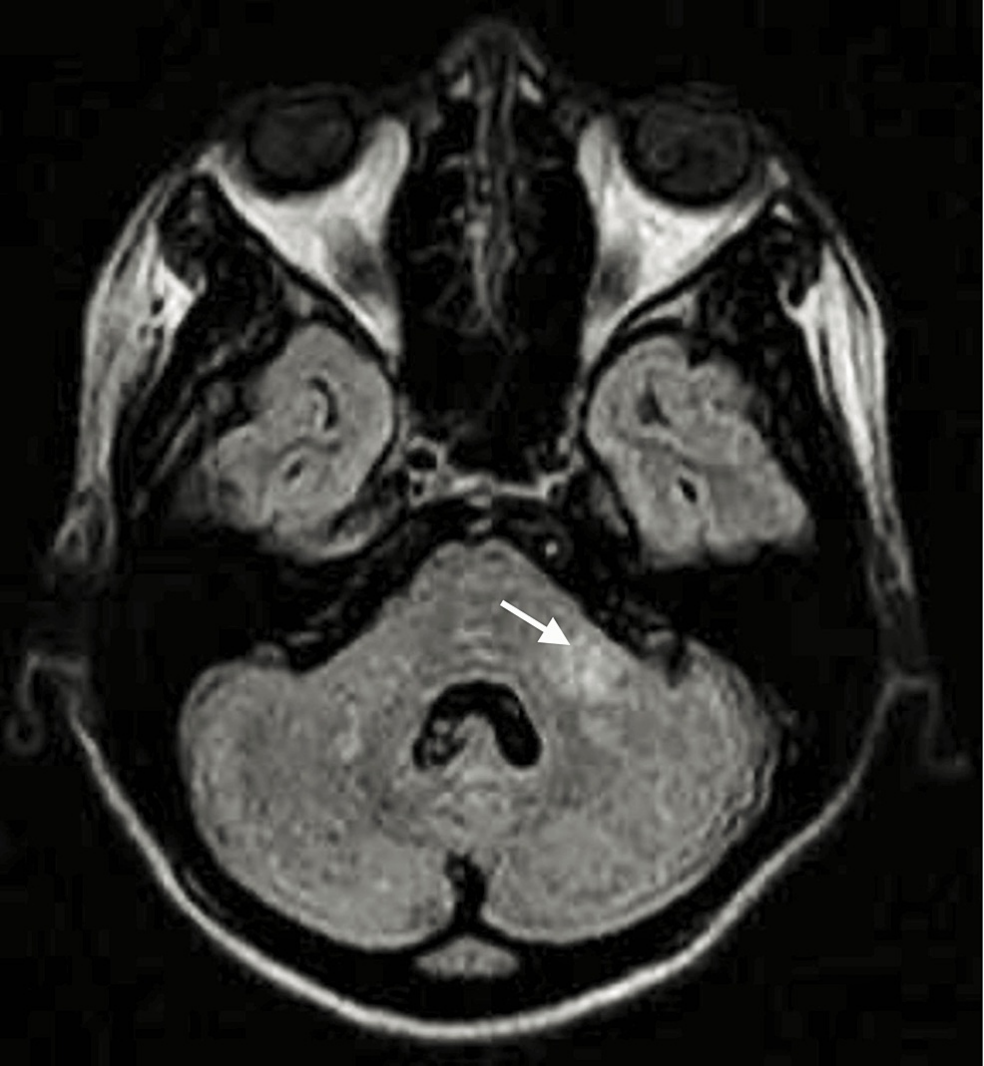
An axial T2-weighted MR image demonstrates hyperintensity (white arrow) in the left cerebellar peduncle
